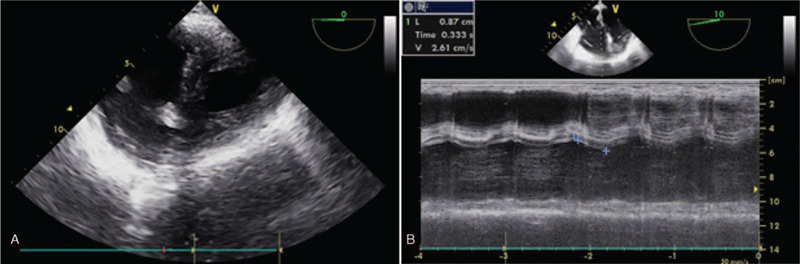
Transesophageal echocardiography findings immediately after the return of spontaneous circulation. In the transgastric mid-papillary short-axis view (A), the right ventricle was seen to be larger than the left ventricle in diastole. M-mode in the mid-esophageal four-chamber view (B) showed a decreased tricuspid annular plane systolic excursion of 8.7 mm (normal range, 15–20 mm).
